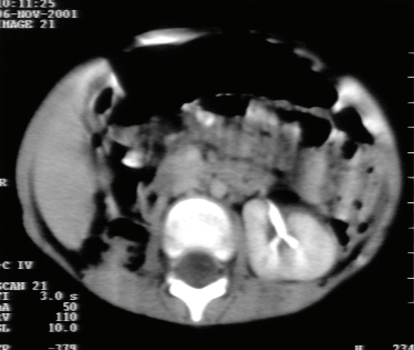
January 2002 - normal abdominal and thoracic CT scans, 2 months after polychemotherapy and radiotherapy.
